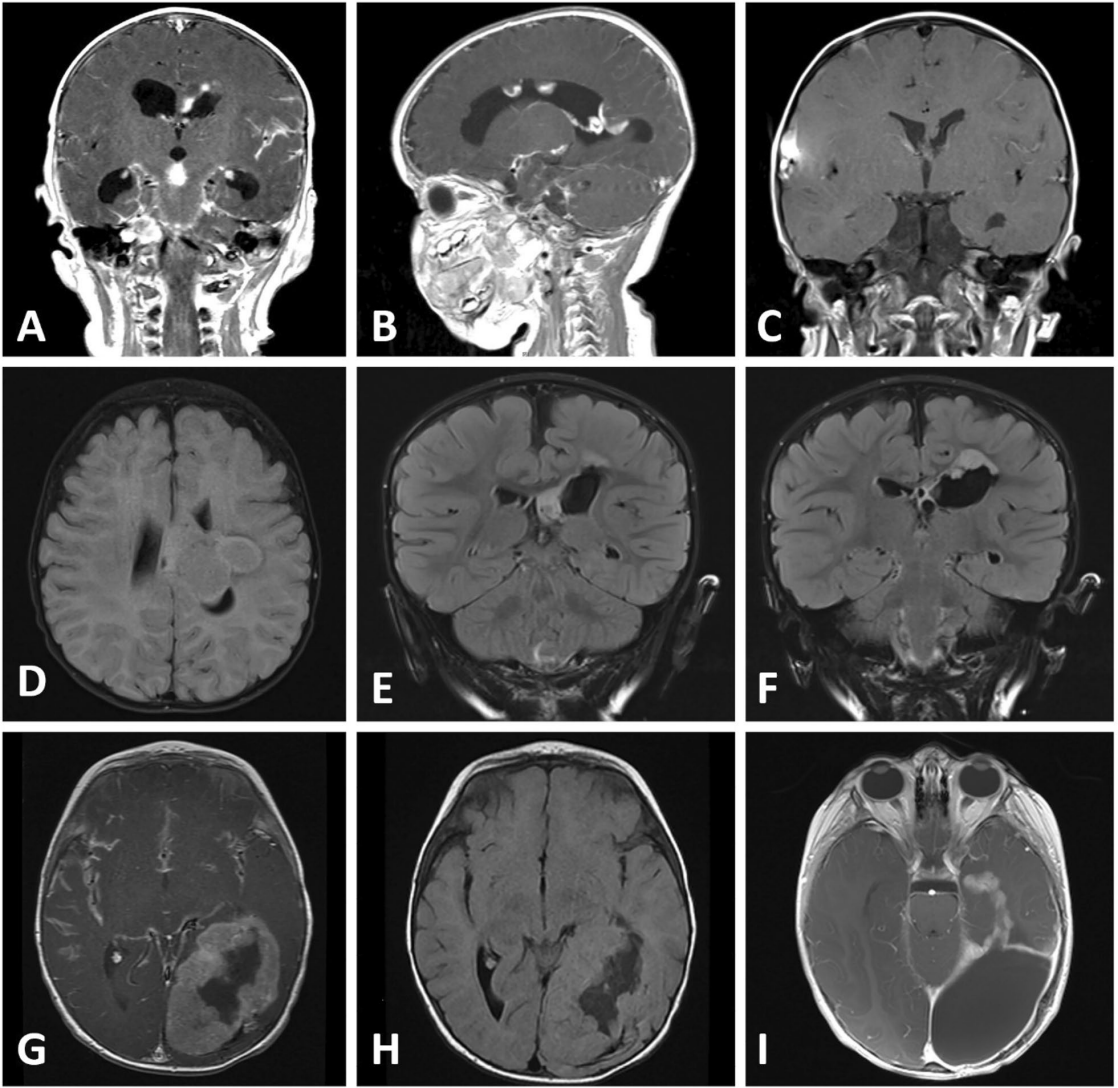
Magnet resonance imaging of the tumors found in the reported cases. A Multiple inhomogeneously contrast-enhancing tumors with a maximum expansion of 10 × 20 mm and B an infratentorial tumor manifestation in the right cerebellopontine angle with extension in the internal auditory meatus were found in the first case. C Follow-up contrast enhanced T1 MRI scan of the first case 3 years after diagnosis showing a stable residual in the right lateral ventricle adjacent to the septum pellucidum without contrast enhancement. D T2 flair MRI scan of case 3 at initial diagnosis showing a large heterogeneous mass arising from the deep white matter of the posterior left frontal lobe with extension into the left lateral ventricle. E and F Coronal T2 flair MRI images of case two attained approximately 2 years after initial diagnosis showing a multinodular recurrence in close proximity to the left lateral ventricle. Contrast enhanced T1 (G) and T2 flair (H) MRI scans of case 3 at initial diagnosis showing a large tumor involving the left parietal, occipital and temporal lobe with extensive intratumoral hemorrhage. I Follow-up MRI scan six months after initial therapy showing a progression of the diffuse contrast enhancing residual tumor
